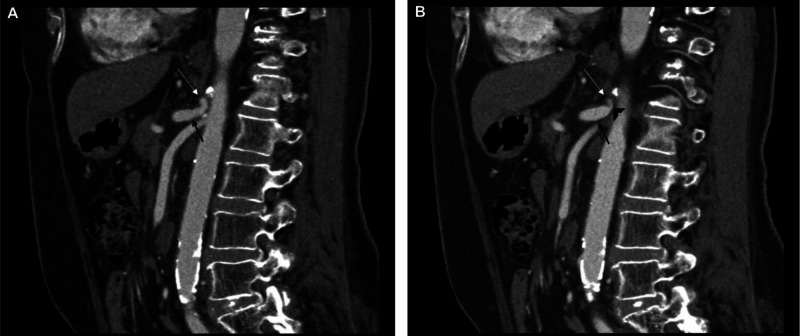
Sagittal CT angiography images of abdomen/pelvis (A, B) demonstrates proximal celiac artery narrowing (white arrows), post-stenotic dilation (black arrows), and (B) characteristic hooked appearance (arrowhead) of the proximal celiac artery
